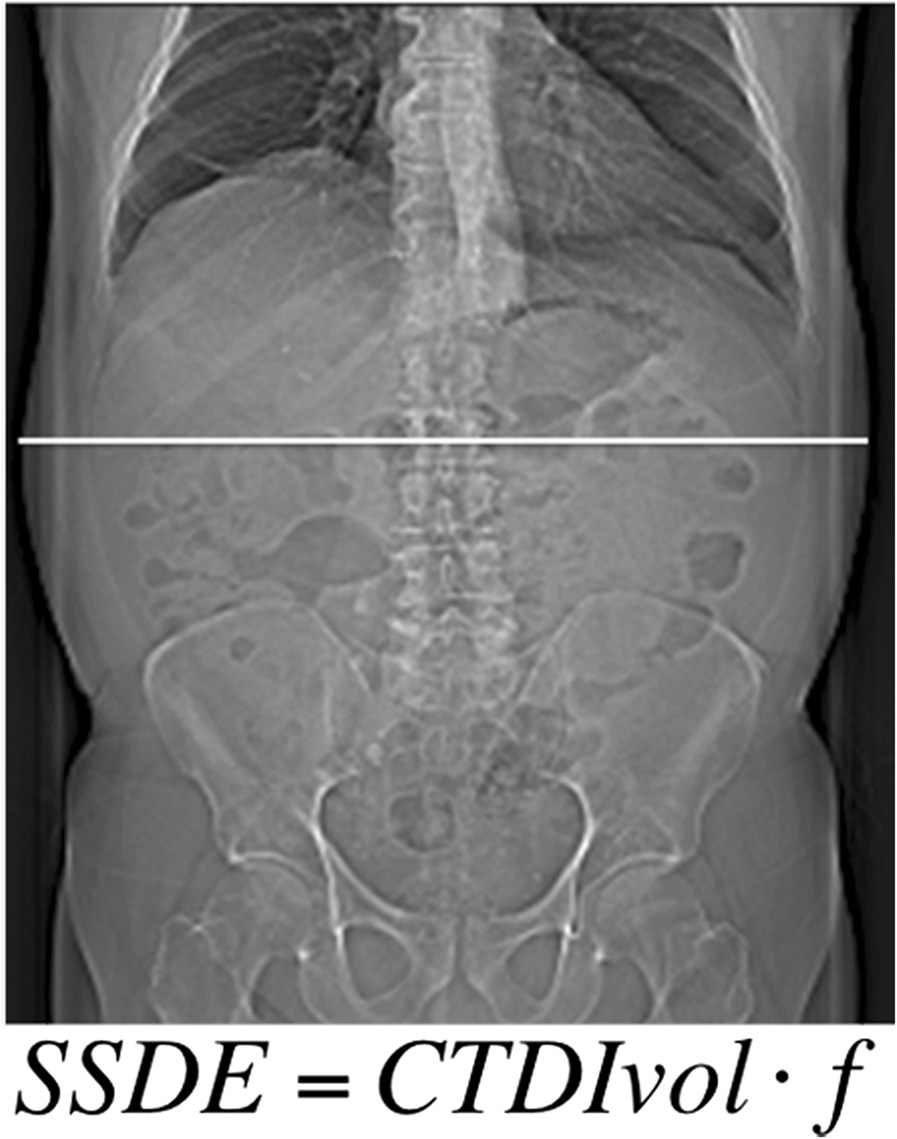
Size-specific dose estimates. Lateral patient diameter was determined on CT-topogram. Based on diameter-dependant conversion factors (f) and CT dose index (CTDIvol), size-specific dose estimates (SSDE) can be calculated
